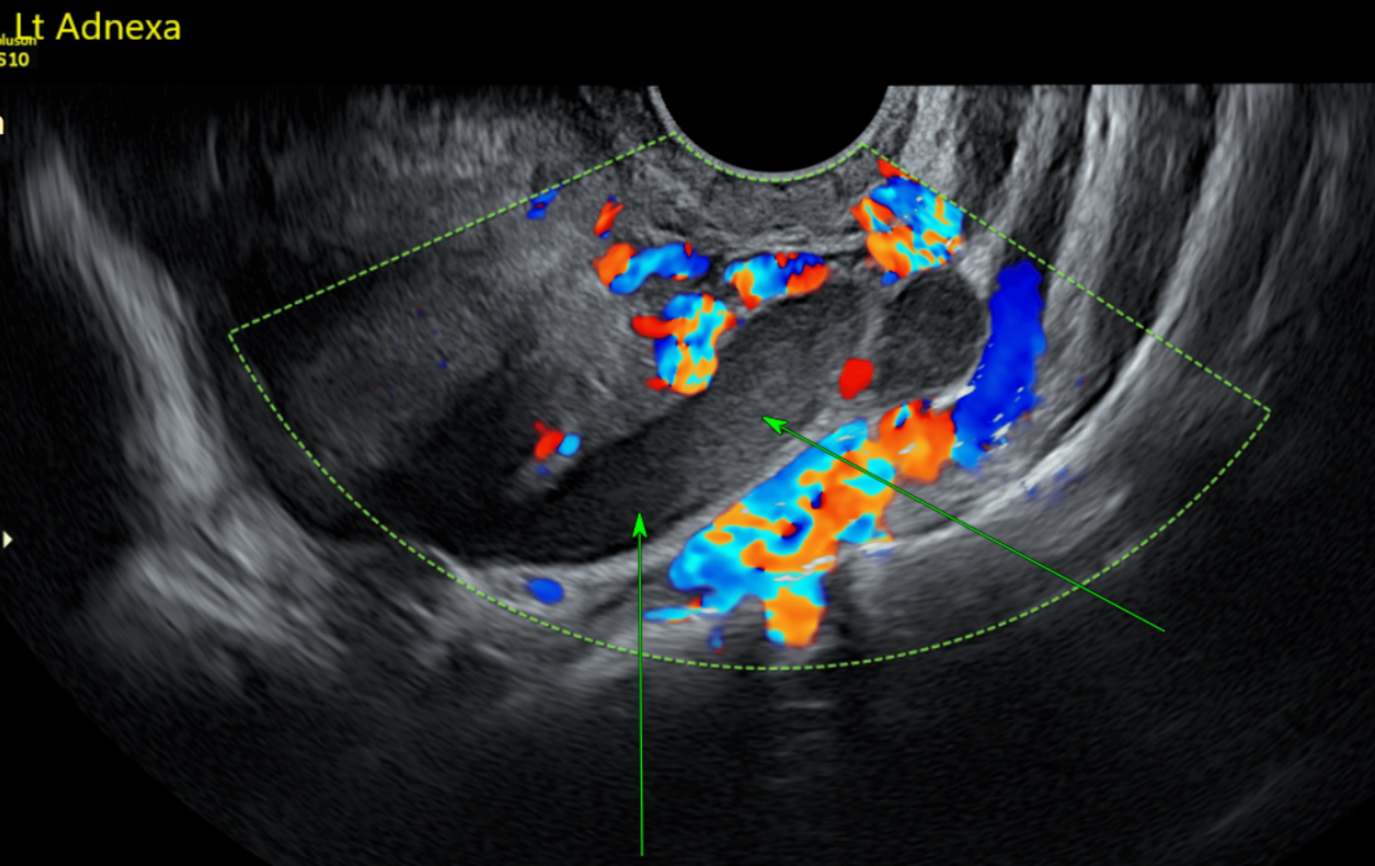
Transvaginal ultrasound showing a tubular structure consistent with a haematosalpinx.
